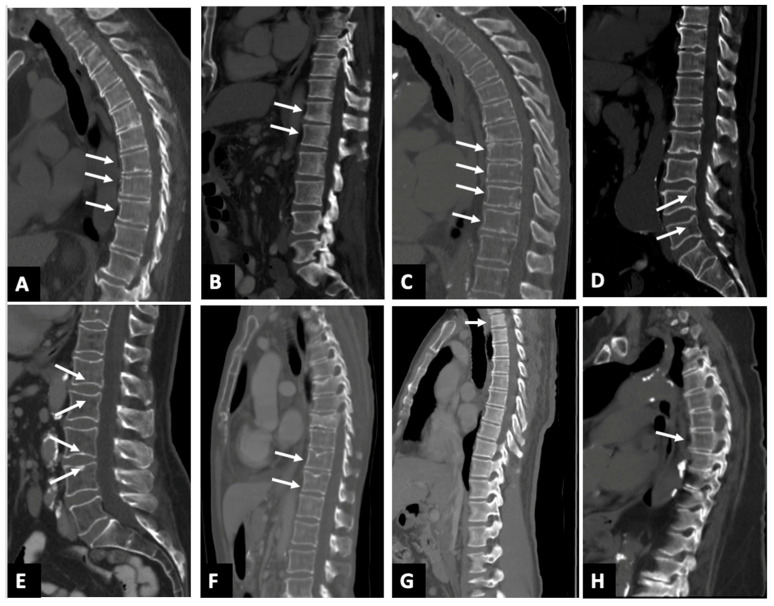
Sagittal CT images (A–H) in 8 different patients with AI software calling false positive fracture. (A) the white arrows show osteoarthritic wedging deformity involving vertebral bodies T8–10; (B) the white arrows show physiological wedging deformity involving vertebral bodies T12–L1; (C) the white arrows show endplate irregularities denoting Scheuermann’s disease, noted in multiple lower thoracic vertebral bodies, namely T8–11; (D) the white arrow shows cupids bow deformity noted in lumbar vertebral bodies L4–L5; (E) the white arrows show concavity/ballooned disk spaces noted in lumbar vertebral bodies L1–L4; (F) the white arrows show Schmorl’s node involving vertebral bodies T11–12. Fractures of T7 and T9 vertebral bodies were accurately identified; (G) the white arrows show edge of field of view overcalls involving the T1 vertebral body that appears normal; (H) the white arrows show T6 fracture overcall in a patient with scoliosis.
